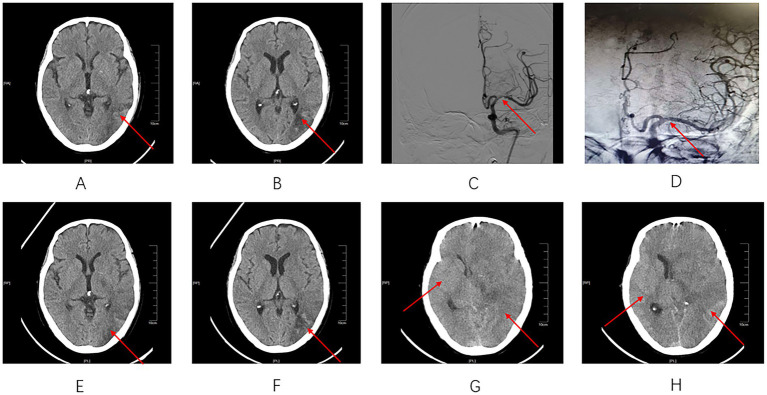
Serial neuroimaging before and after mechanical thrombectomy. (A) Axial CT shows a large hypodense area in the left fronto-temporo-parietal region (arrow), consistent with an extensive infarction in the left MCA territory. No obvious midline shift is observed at this stage. (B) A slightly higher axial level again demonstrates low attenuation in the left cerebral hemisphere with cortical sulcal effacement (arrow), but no obvious midline shift at this stage. (C) Left internal carotid angiogram shows distal MCA occlusion/severe stenosis with poor distal filling (arrow). (D) Post-thrombectomy angiogram demonstrates restored antegrade flow in the left MCA with re-opacification of distal branches (arrow). (E) Axial CT shows increased edema of the infarcted left cerebral hemisphere with sulcal effacement. A focal hyperdense area is seen within the infarct zone (arrow), suggesting contrast extravasation or mild hemorrhagic transformation in the early post-reperfusion stage. (F) At a slightly different level, the same linear/wedge-shaped hyperdensity is again demonstrated (arrow), accompanied by compression of the left lateral ventricle and a mild rightward midline shift, indicating a vulnerable blood–brain barrier after endovascular therapy. (G) Diffuse swelling of the left cerebral hemisphere with effacement of the cortical sulci, marked compression of the left lateral ventricle, and rightward midline shift. (H) Further progression of hemispheric edema with near-complete obliteration of the left lateral ventricle and a marked nonlinear increase in rightward midline shift to 11 mm within 20 h of the previous scan, consistent with malignant cerebral edema and impending brain herniation.
